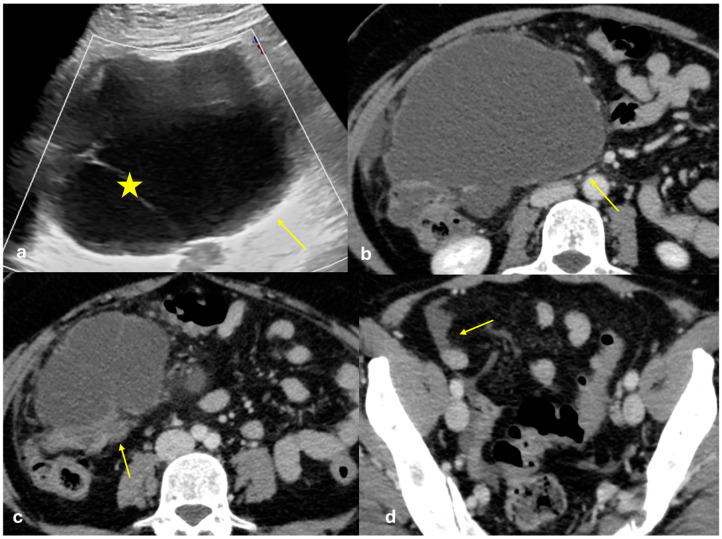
Mesenteric pseudocyst. A middle-aged woman with abdominal pain and a palpable mass in the right hypocondrium. US reveals a large cystic anechoic mass of unknown origin located next to the liver hilum with a thin nonvascularized septum ((a), arrow; star on the septum). CE-CT shows a large cystic mass located anteriorly to the ascending colon ((b,c): arrows). A small amount of fluid which may be secondary to cyst rupture is visible in the pelvis ((d): arrow).
